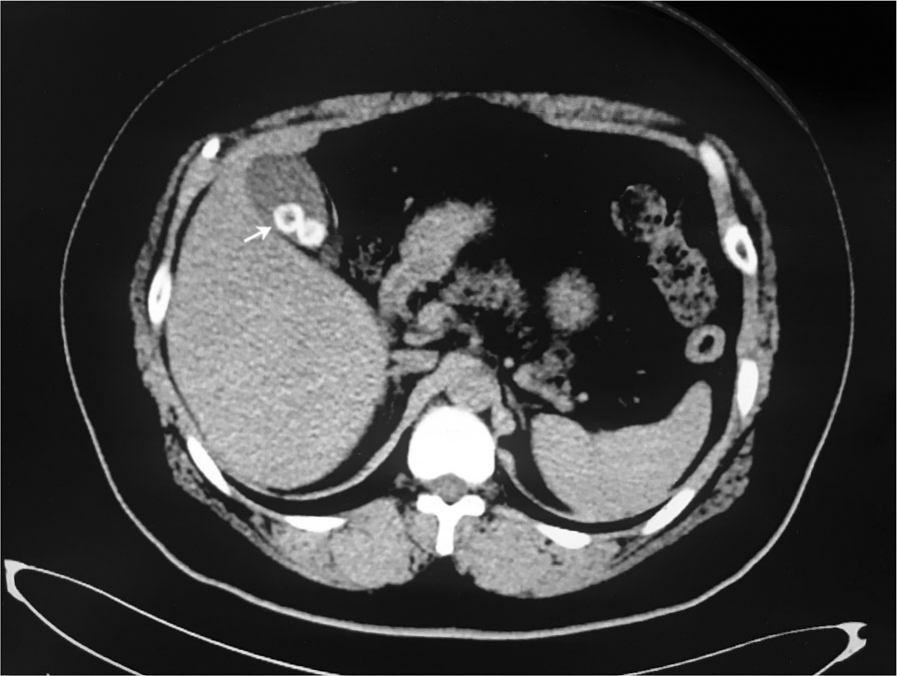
CT image showing stone-like substance in the gallbladder (white arrow)
